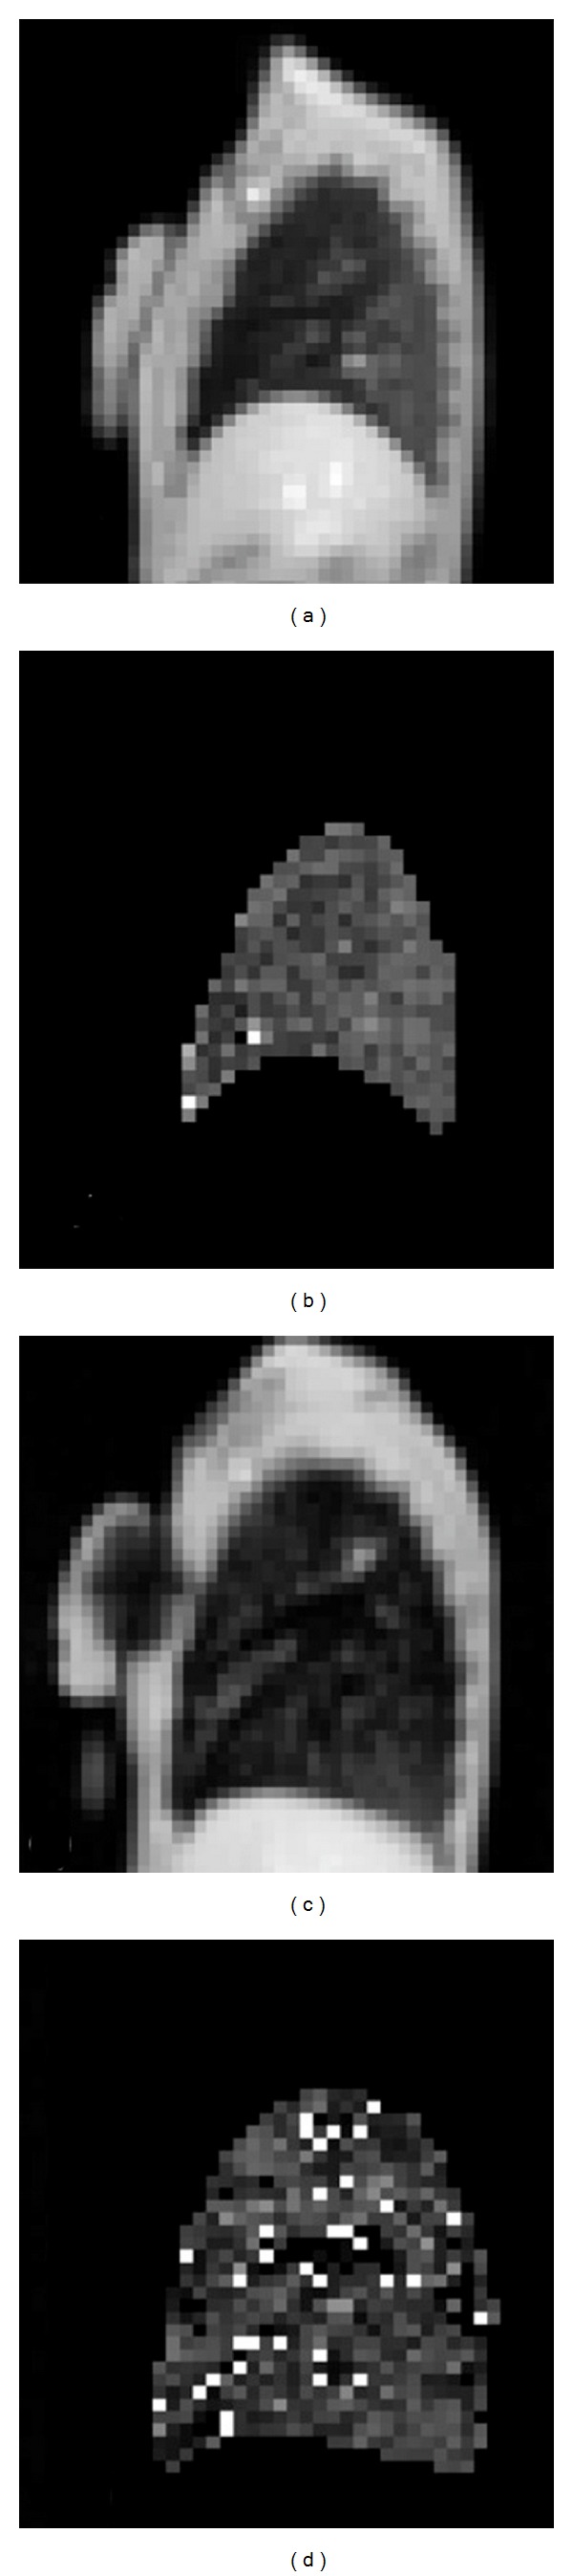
Image data (a, c) and corresponding lung density maps (b, d) for a healthy male (41 yo, FEV1[pred] = 106% (a, b)) and a CF male with severe disease (42 yo, FEV1[pred] = 37% (c, d)) using an MRI methodology as described in a study conducted by Theilmann et al. [6]. The artifact in the chest wall in (d) is due to a metal ring in an installed port-a-cath. The hyperintense regions in the lung density map of the CF subject (d) indicate lung regions full of mucus and regions showing an absence of water are associated with tissue scarring. Neither observation is seen in the healthy subject.
